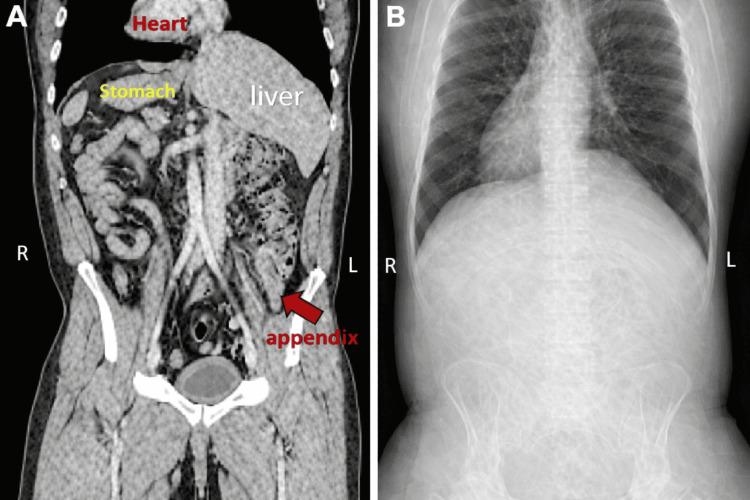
(A) Coronal abdominal CT image demonstrating the cecum and appendix on the left side. (B) Chest radiograph demonstrating a right-sided cardiac apex (dextrocardia).
